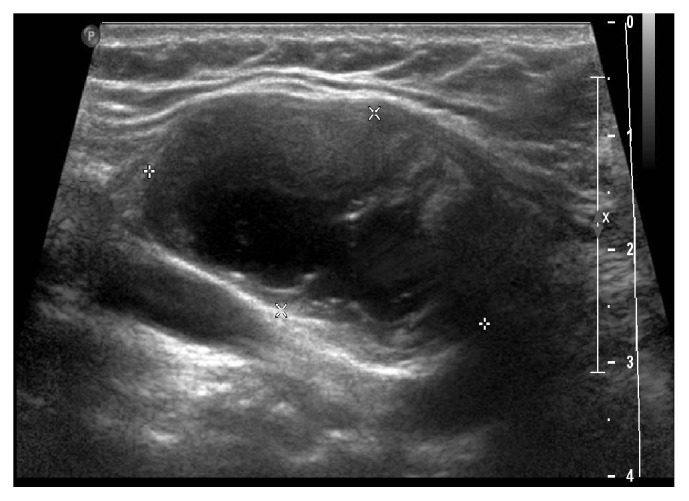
Right-sided dominant thyroid nodule seen on thyroid ultrasonography.
